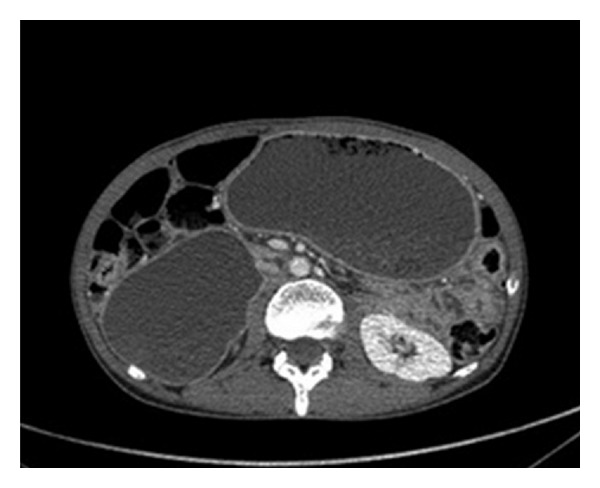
Abdominal computed tomography showing gastric and duodenal distension related to an irregular thickening with stenosis localized to genus inferius duodenal portion.
